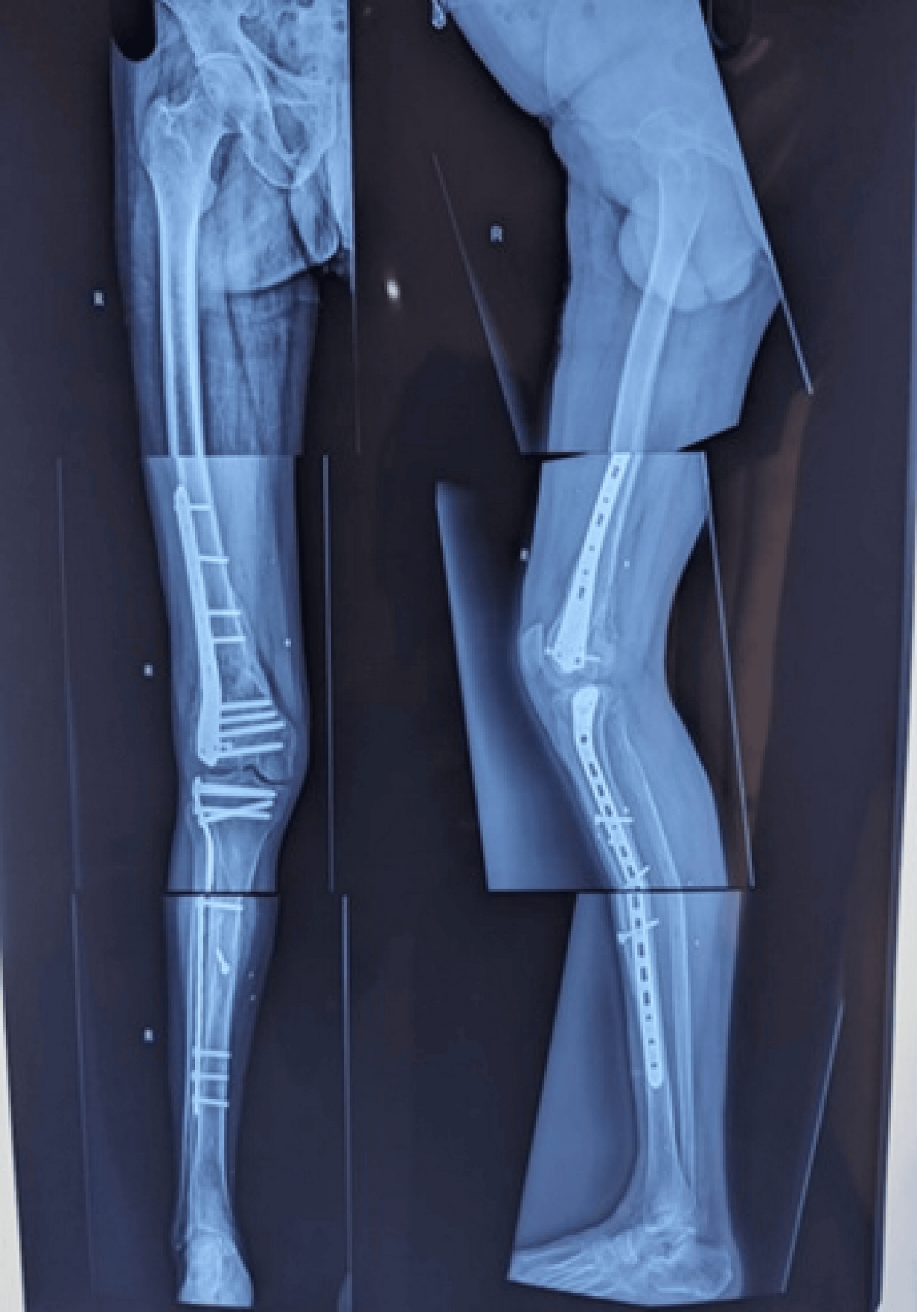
One-Year Postoperative Follow-Up Radiograph of Fraser Type II-C Floating Knee Injury (Closed Type), Showing Satisfactory Alignment and Evidence of Fracture Healing.
